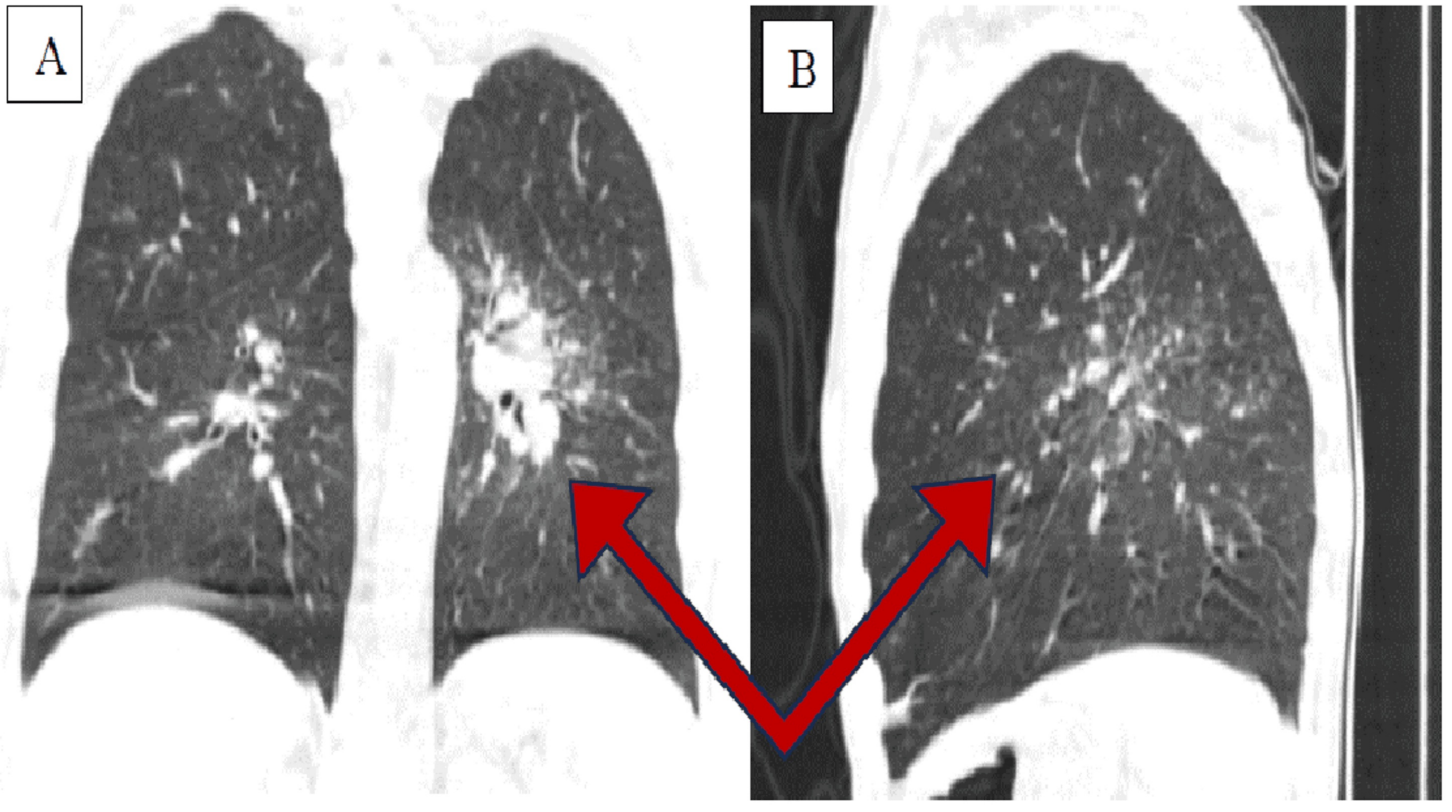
Computed tomography (CT) scan of patient’s lungs showing disseminated nodular focci: (A) front view; (B) side view
